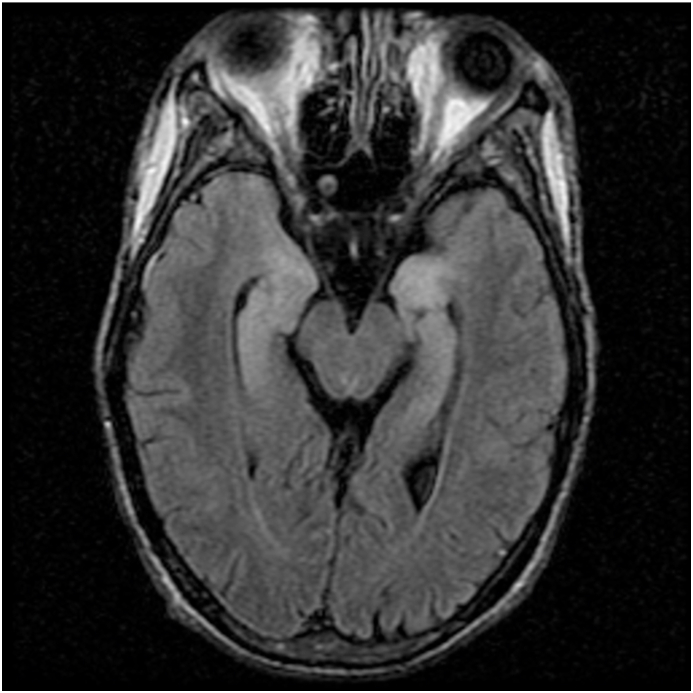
MRI-FLAIR of patient 2; showing hyperintensity of bilateral mesial temporal areas including unci/hippocampi. FLAIR: Fluid-Attenuated Inversion Recovery.
